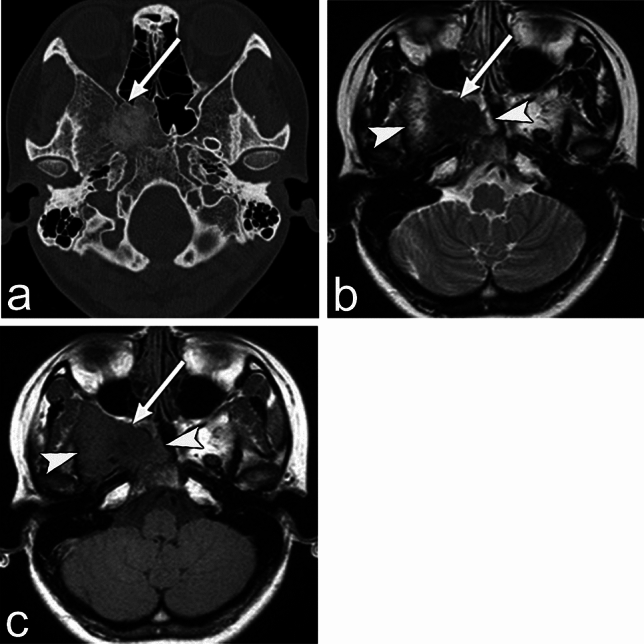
Fibrous dysplasia of an asymptomatic woman in her 20s. The lesion is identified incidentally. a CT shows a ground-glass appearance (arrow) with swelling of the right side of the sphenoid bone. b, c The area (arrow) of the lesion that shows a ground-glass appearance on CT shows low signal intensity on the T2 weighted image (WI) and T1WI. Other areas (arrowheads) of the lesion show high signal intensity on T2WI and mildly high signal intensity on T1WI
